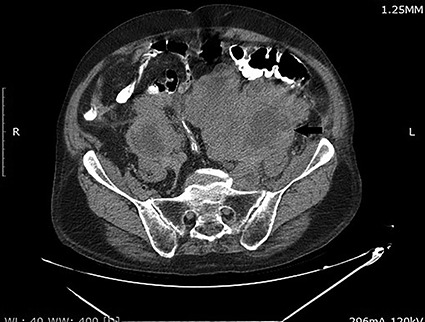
CT scan of the abdomen and pelvis demonstrating a large retroperitoneal mass (arrow).
